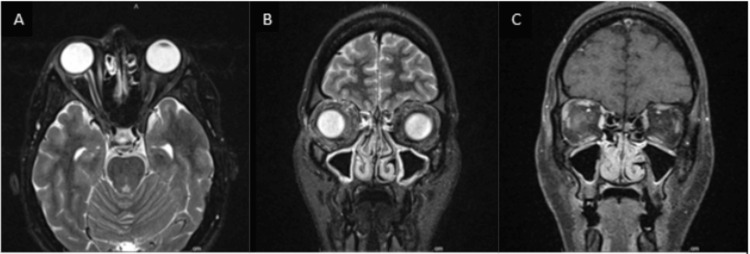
MRI imaging of the head The image shows that the right globe is intact, but there is mild right exotropia present. Subtle edema is present along the superior and lateral contour of the preseptal right orbit, which is best appreciated in the axial STIR images (A). There is a suspected mild extension of edema into the extraconal postseptal fat as in the coronal STIR image (C). Additional STIR signal abnormality with associated mild postcontrast enhancement is present along the posterior contour of the globe lateral to the optic nerve (A). There is mild asymmetric dilation of the right optic nerve sheath with flattening of the optic disc. There is suspected subtle hyperenhancement of the right optic nerve sheath as in the coronal image (B) immediately posterior to the globe. Abnormal T2 hyperintensity and hyperenhancement are present in the right superior and medial rectus muscles best seen in the coronal STIR image (C) and coronal postcontrast image (B). There is no drainable abscess identified, and no pathologic signal or pathologic enhancement is detected in the optic nerve proper. The cavernous sinuses enhance symmetrically STIR: short TI inversion recovery
